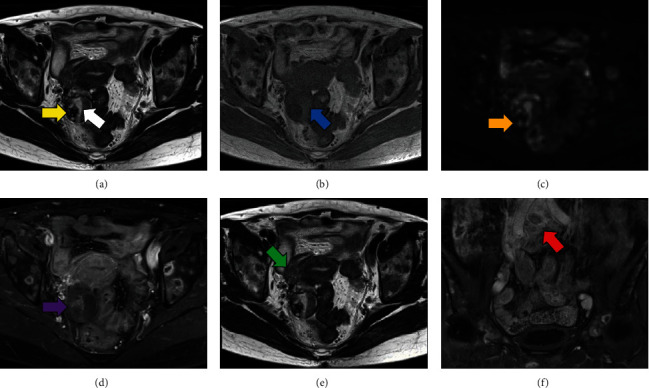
MRI findings. (a) MRI shows a 50 mm multicystic tumor with an internal nodule 15 mm in diameter in the right ovary. The fluid on the dorsal side of the tumor indicates high intensity (white arrow), and the nodule with multiple cysts shows low intensity (yellow arrow) in T2-weighted image. (b) The fluid on the dorsal side of the tumor indicates high intensity in T1-weighted image (blue arrow). (c) DWI shows partially reduced diffusion of the nodule (orange arrow). (d) Contrast-enhanced imaging shows heterogeneously enhanced contrast of the tumor (purple arrow). (e) The nodule in the right round ligament in T2-weighted image (green arrow). (f) Swollen lymph nodes of the pelvis in contrast-enhanced T1-weighted imaging (coronal plane, red arrow). MRI: magnetic resonance imaging; DWI: diffusion-weighted image.
